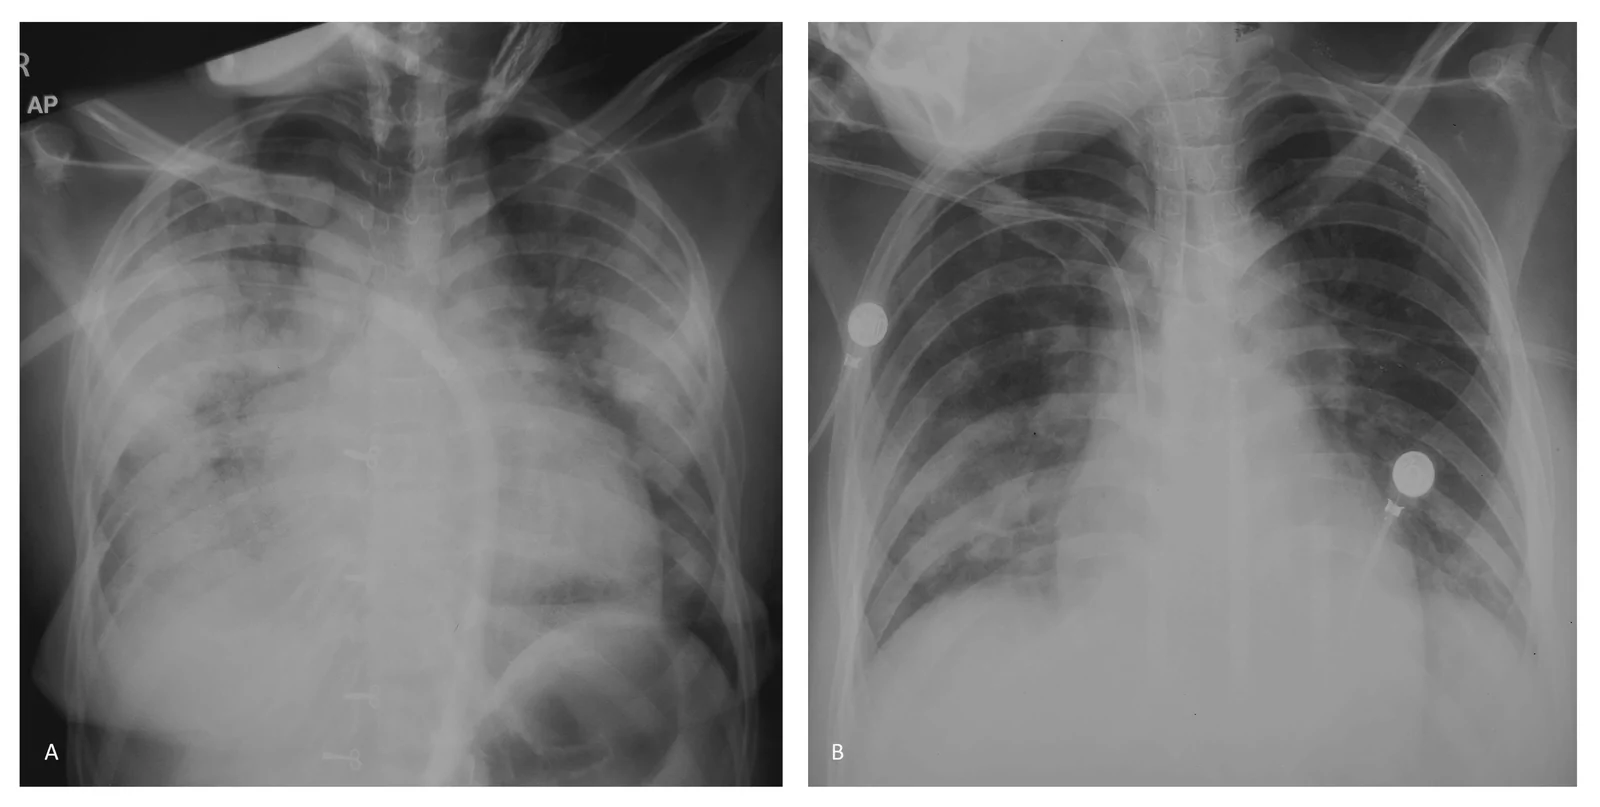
X-ray Chest AP view A: Bilateral fluffy airspace opacities suggestive of acute respiratory distress syndrome visible on day 1 of hospitalisation; B: Significant resolution of opacities visible on day 4 of hospitalisation.
